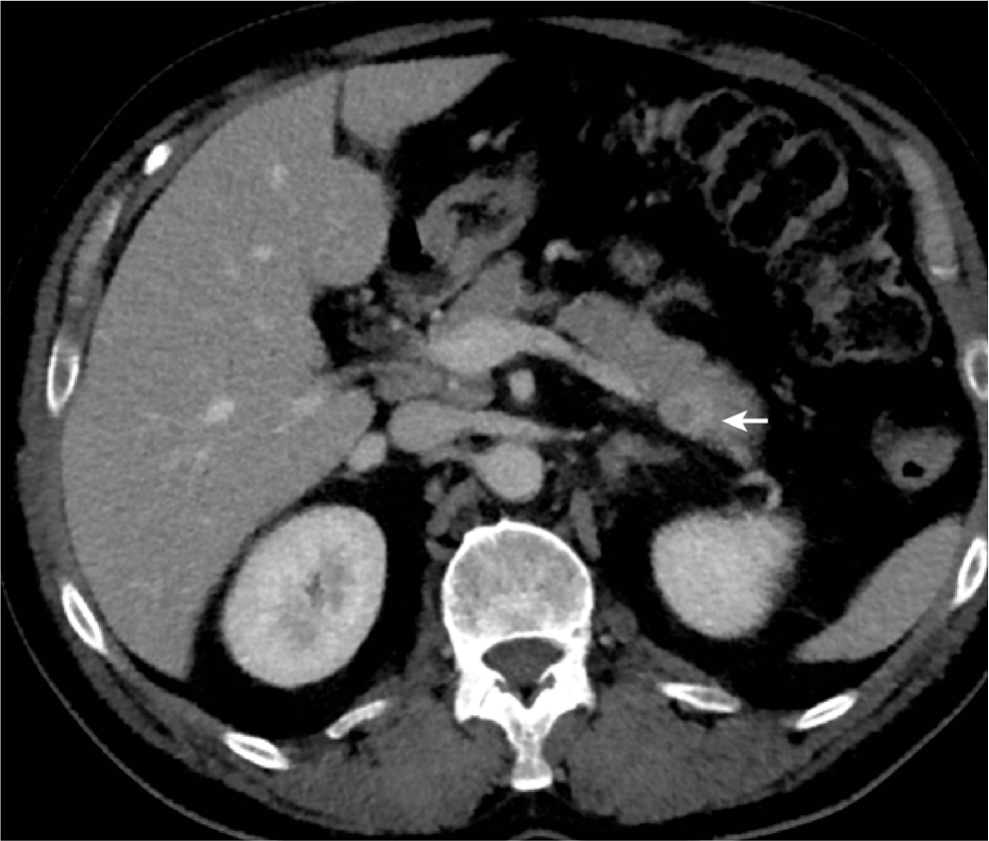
Abdominal computed tomography (*CT*) shows a 15 × 12-mm tumor (*white arrow*), which is heterogeneously enhanced by contrast media
